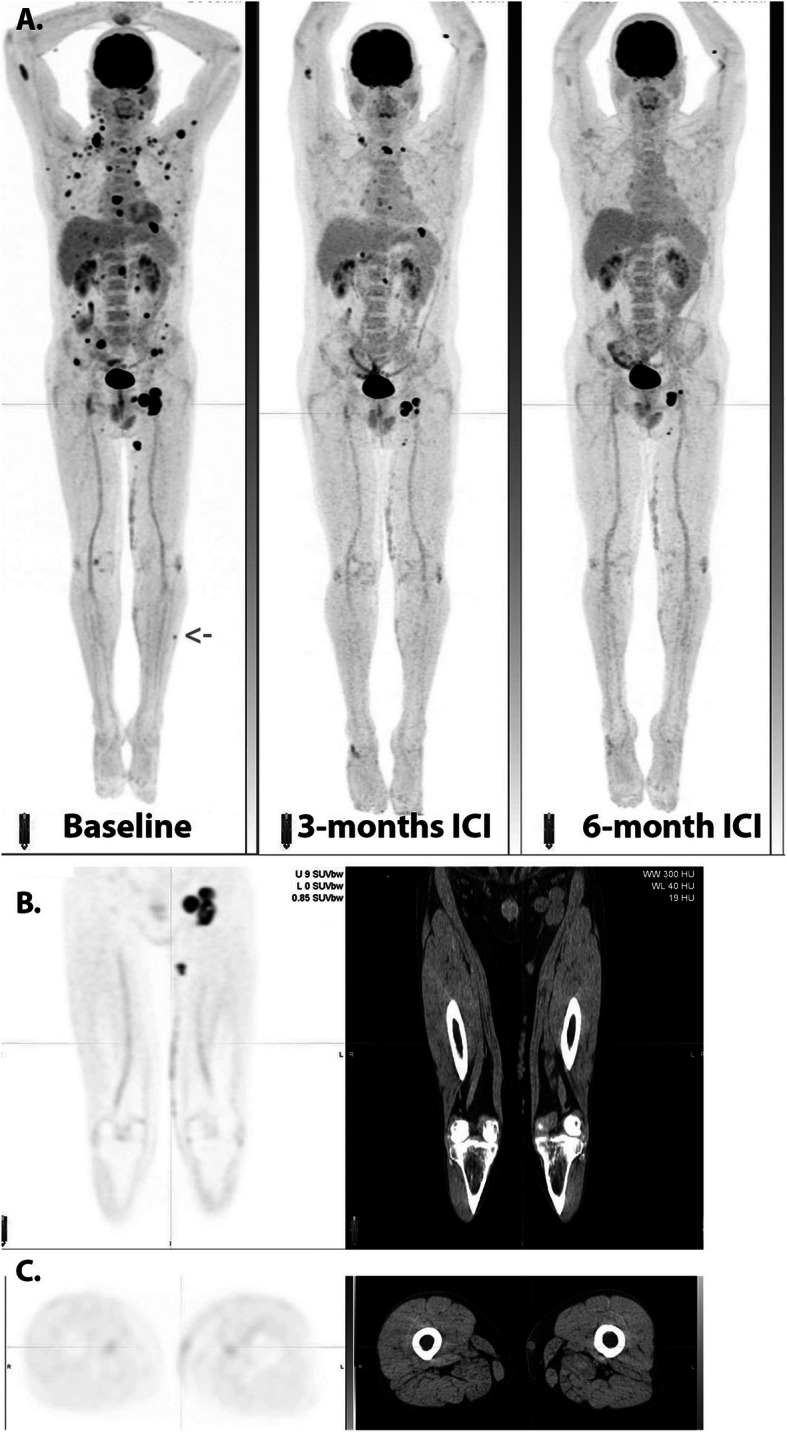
Serial MIP images (A) in a patient with widespread metastatic melanoma demonstrate resolution of an in-transit deposit on the lateral aspect of the left leg (arrow on baseline scan) and gradual regression of metastatic disease over time despite residual left inguinal nodal abnormality after 6 months of immune checkpoint therapy. On coronal PET and CT images (B), nodular but contiguous linear uptake in the subcutaneous fat along the medial left thigh demonstrates no change over time on the MIP images above and clinically correlated with a varicose vein. Transaxial images (C) are more difficult to interpret than the coronal images in demonstrating mildly ^18^F-FDG -avid nodularity and a rounded shape on correlative CT, emphasizing the importance of review of images in orthogonal planes
